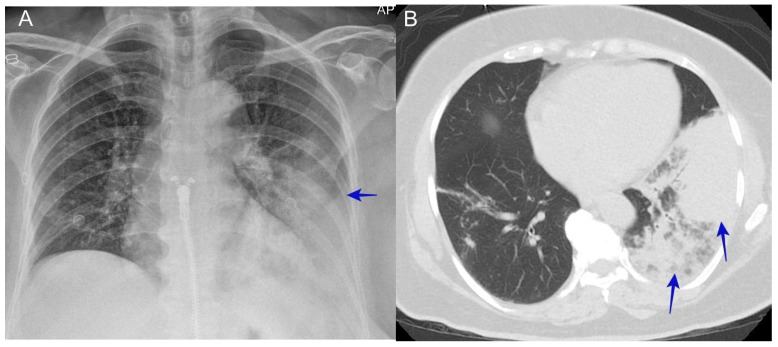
(A) A chest X-ray shows a left lower opacity (blue arrow). (B) A CT scan shows left lower lobe consolidation (blue arrows). The patient was a 65-year-old woman who presented with the chief complaint of a cough and dyspnea lasting for one week. She was diagnosed with community-acquired pneumonia and placed on IV antibiotics and sent home with oral antibiotics. She tested positive for coccidioidomycosis IgG.
